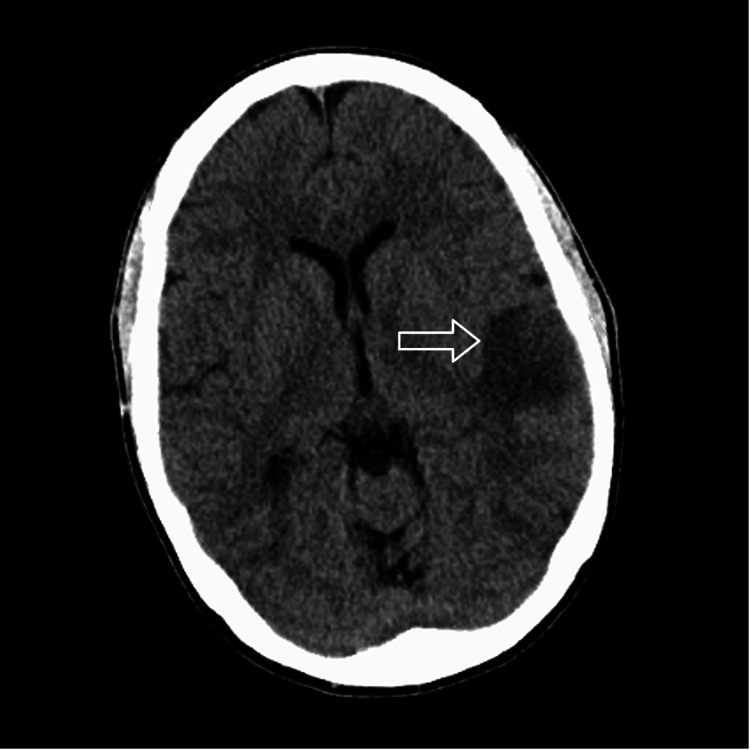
Head CT scan without contrast revealing subcortical hypodensity in the left temporal region with adjoining cortical sulci effacement.
